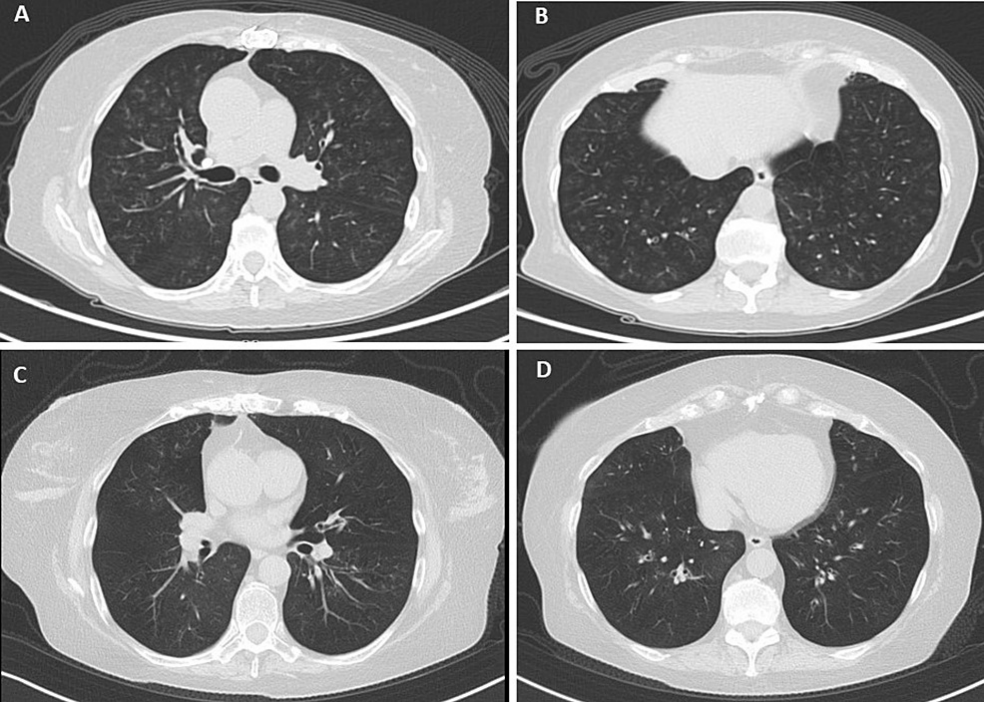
CT scan for Patient 2 A-B: The CT scan shows centrilobular ground glass nodules with tree-in-bud pattern in the upper and lower lobes of both lungs. C-D: Control CT scan shows improvement in parenchymal compromise after mepolizumab was started. The ground glass centrilobular nodules disappeared.
